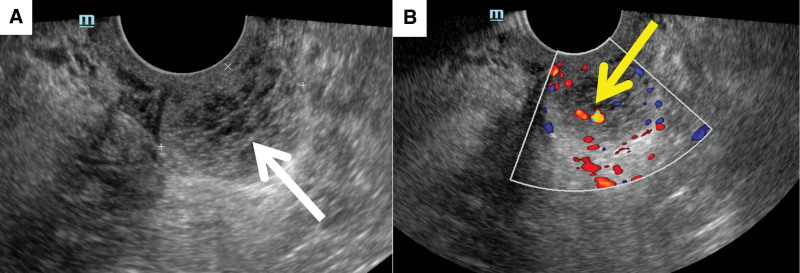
Transvaginal color Doppler ultrasonography. (A) A systic-solid mixed echo mass (white arrow) in the posterior vaginal wall area, with a size of about 30 × 23 × 26 mm, with regular shape and clear boundary. (B) Color Doppler ultrasound showing a dotted and streaky blood flow signal (yellow arrow) at its internal lesion.
